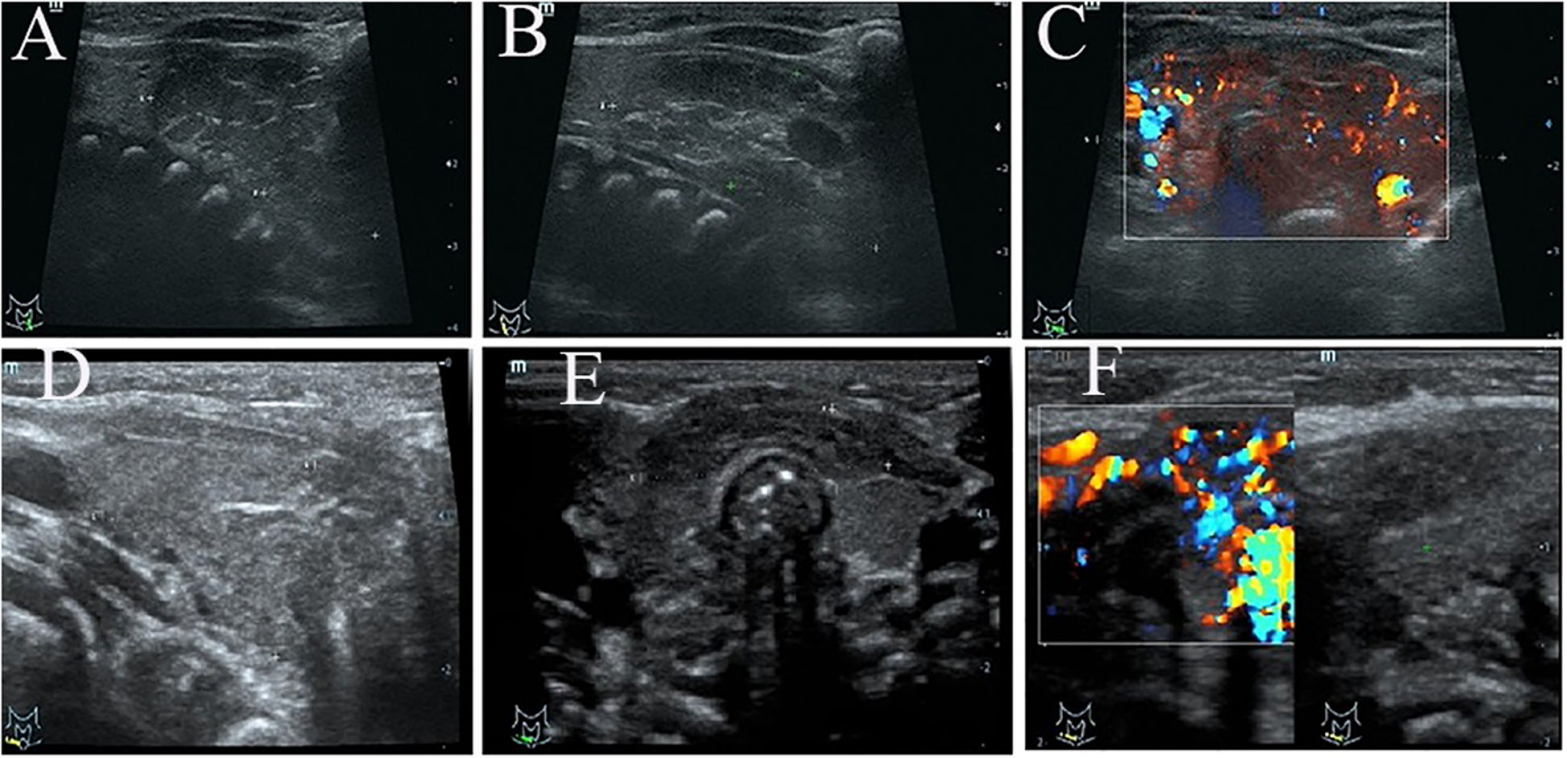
Ultrasound image of thyroid hemangioma. a Hypoechoic lesions are observed at the lower pole of the left thyroid and a chord-like hyperechoic lesion is observed within. b Hypoechoic lesions are observed at the lower pole of the right thyroid, and a chord-like hyperechoic lesion is observed within. c Hypoechoic lesions with a clear boundary and abundant blood supply are noted at the lower pole of the bilateral thyroid. d, e, f No recurrence of hemangioma was found
